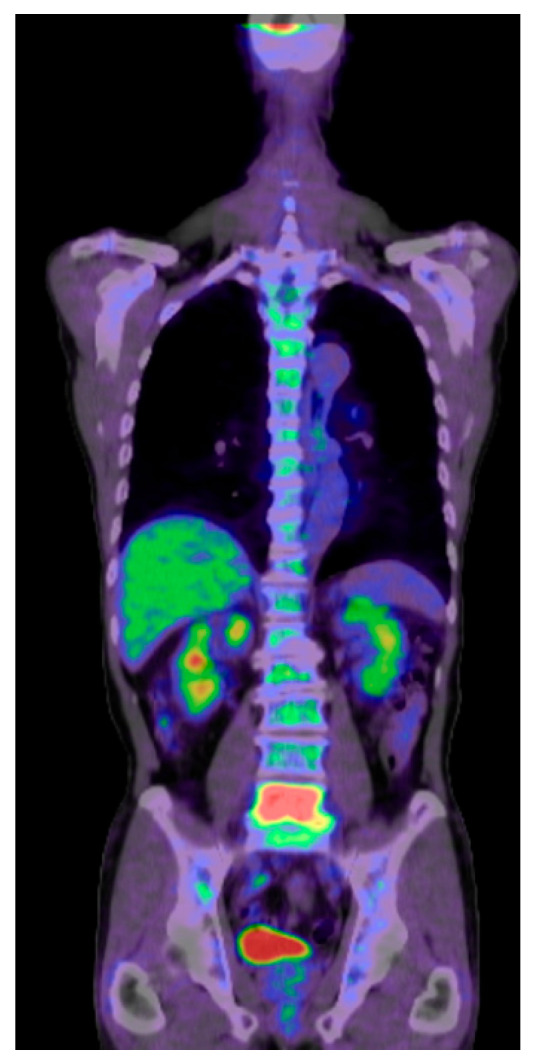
Seventy-two-year-old M, metastatic spinal tumor from maxilla cancer, PET CT, maxilla, and L5 are high uptake.
